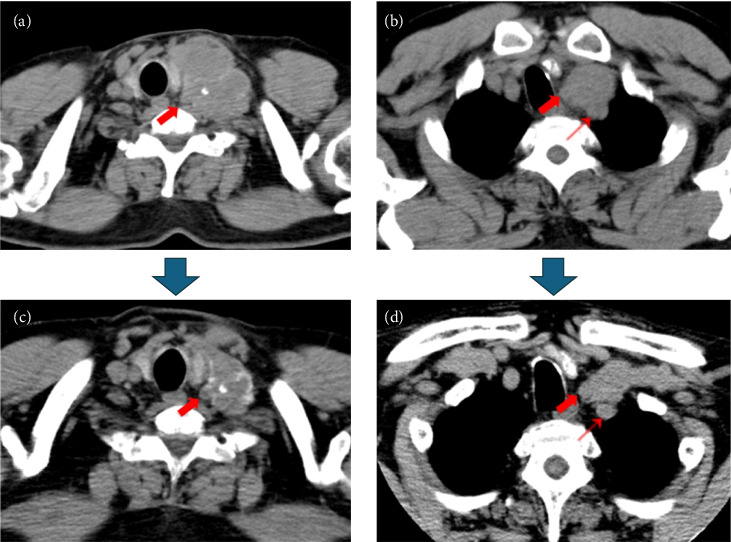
Computed tomography findings before and after neoadjuvant chemotherapy with selpercatinib. The left supraclavicular mass invading the left common carotid artery (➡) and left subclavian artery (→) before the neoadjuvant chemotherapy (a, b). The tumor shows shrinkage upon treatment, and the major axis shows reduced diameter from 77 to 58 mm. Furthermore, the tumor has receded from the left common carotid and left subclavian arteries (c, d).
